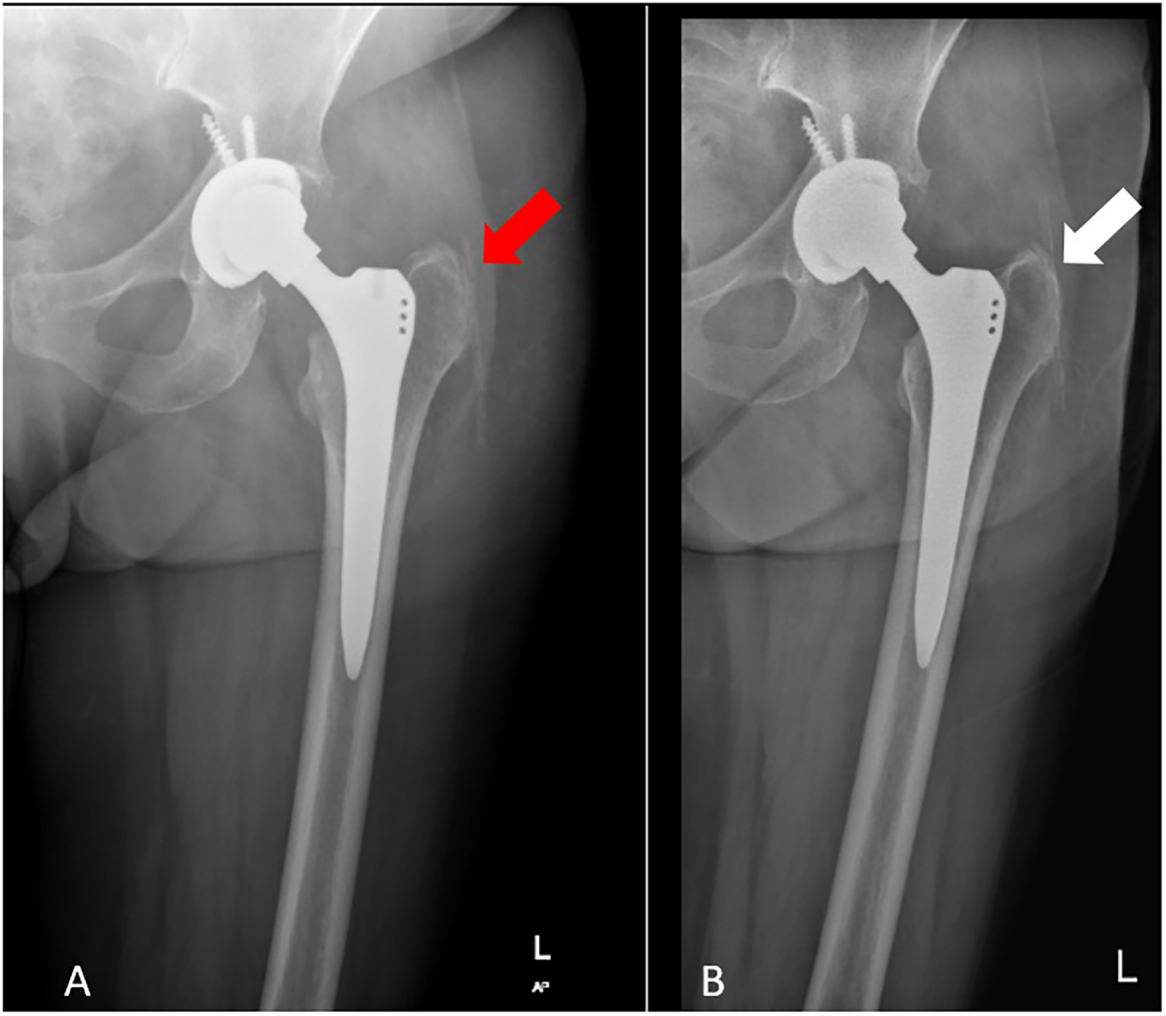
(A) Initial postoperative radiograph showing misdiagnosed retained drain (limited view). (B) X-ray showing the retained drain fragment two years post-surgery.
